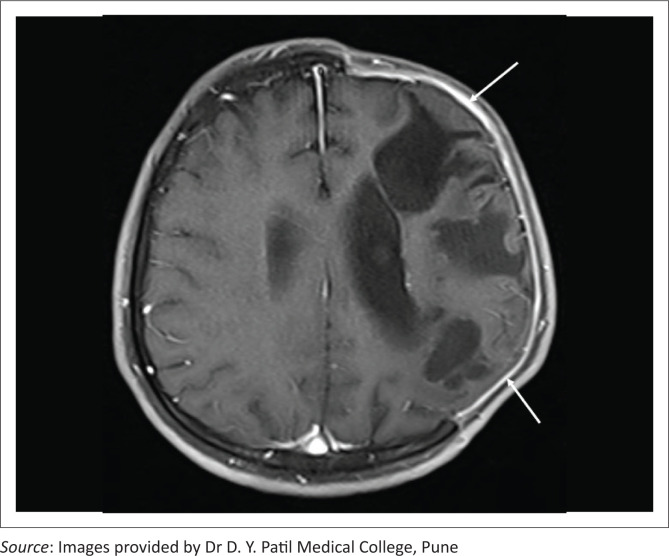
Axial post-contrast T1 weighted MR image in a patient with a left fronto-parietal decompressive craniectomy demonstrates herniation of the brain parenchyma through the craniectomy site along with a thickened enhancing meningogaleal complex (white arrows).
